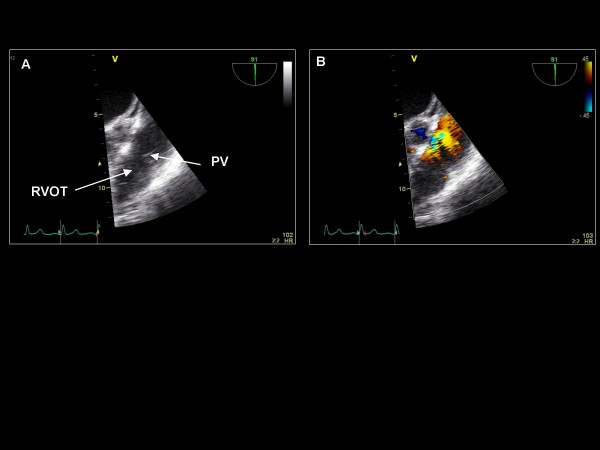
Imaging plane 90°, (A) The right ventricular outflow tract (RVOT) and pulmonic valve (PV). (B) Color Doppler is applied to the pulmonic valve demonstrating trivial pulmonic regurgitation.
